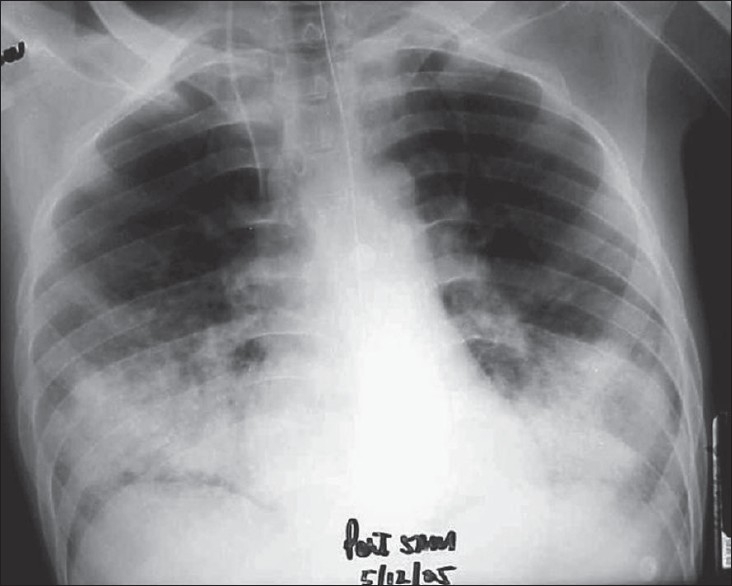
AP radiograph of chest showing bilateral basal air space filling lesions consolidation
